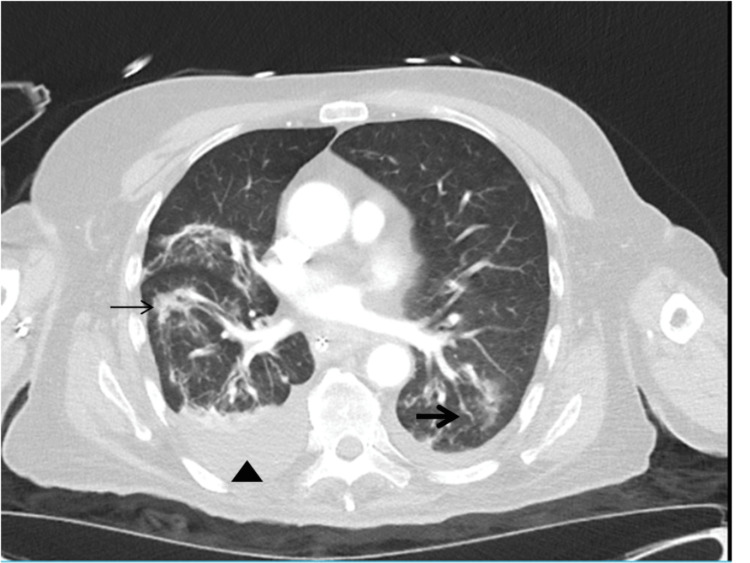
CT scan showing multilobular lung infiltration. CT chest scan showing diffuse, patchy, ground-glass, and nodular pneumonic infiltrates in the right lung in the upper middle and lower lobes (thin arrow), and mostly lower lobe, patchy, ground-glass, nodular infiltrates with consolidation in the left lower lobe (thick arrow). There is a small right pleural effusion (triangle).
